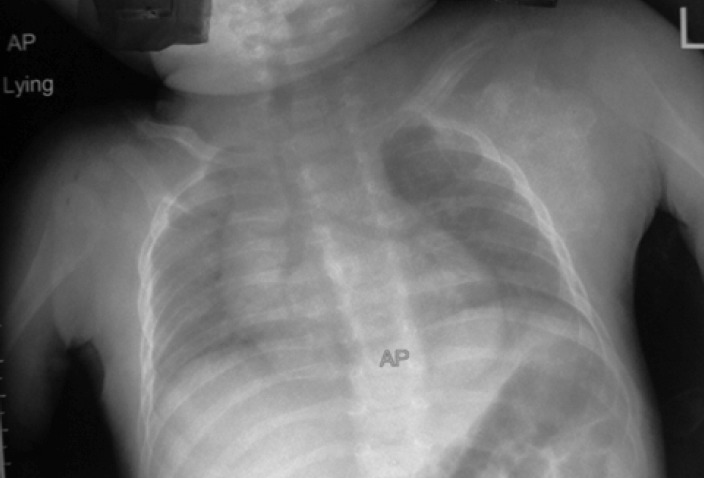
Initial anteroposterior radiograph of the chest and both
